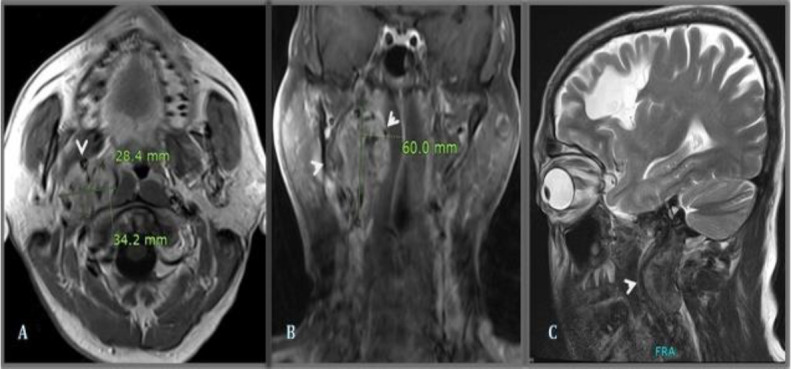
Pre-treatment MRI head and neck examination with IV gadolinium contrast. (A) T1 weighted post-contrast [axial section], (B) T1 weighted post-contrast [coronal section] and (C) T2 weighted [sagittal sections], shows an abnormal signal inten
